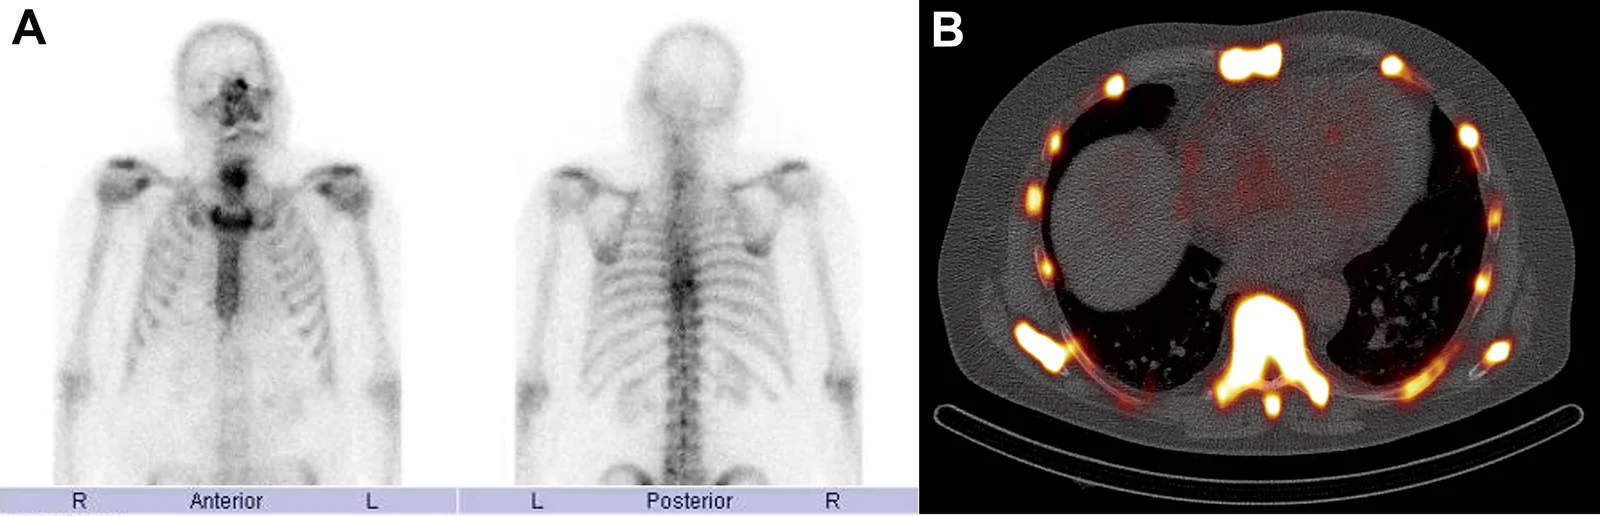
Bone Scintigraphy Planar imaging (A) and single-photon emission computed tomography (B) did not show any cardiac uptake (Perugini grade 0) on technetium-labeled bone scintigraphy.
